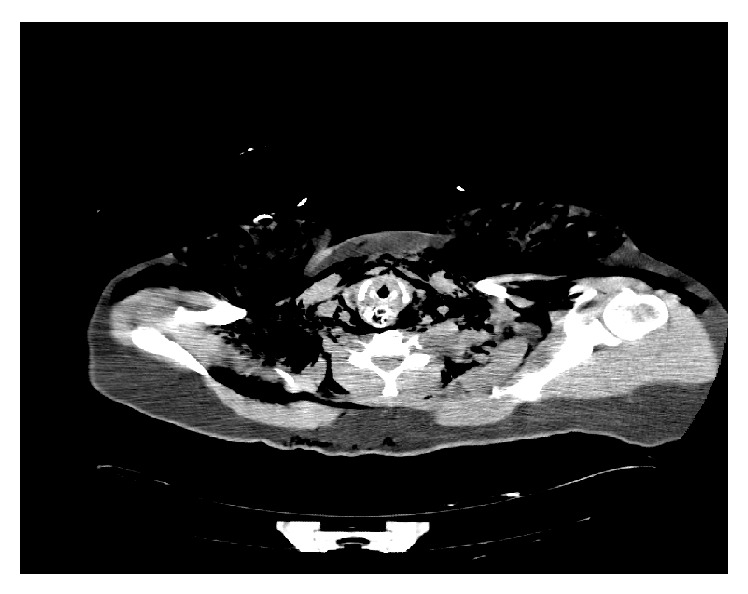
CT scan axial section showing the foreign body and subcutaneous emphysema.
